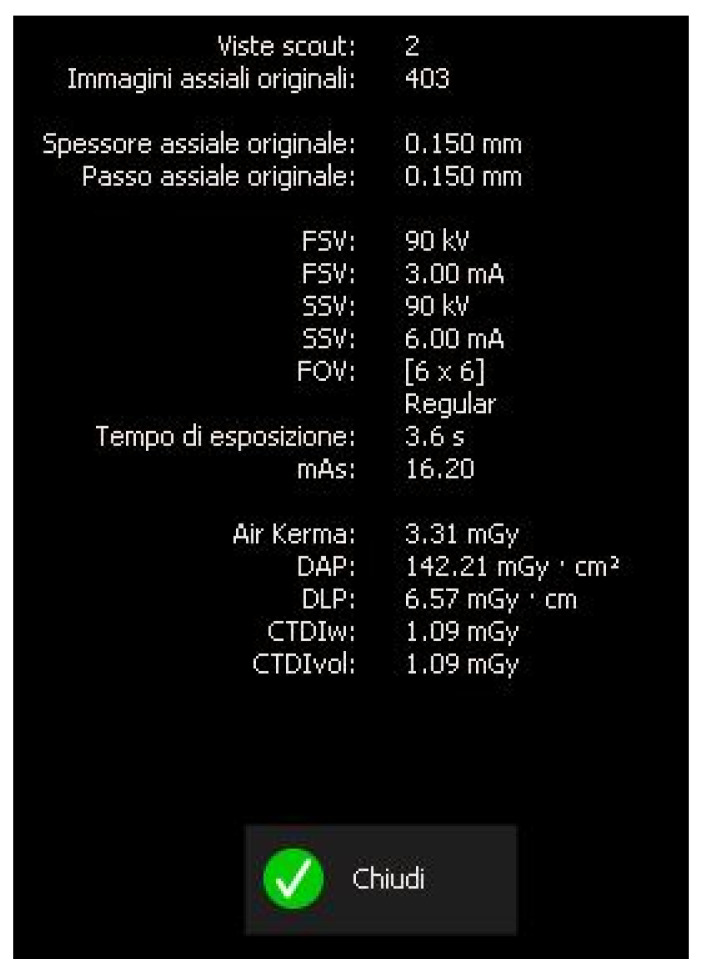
Exposure parameters of CBCT image.
